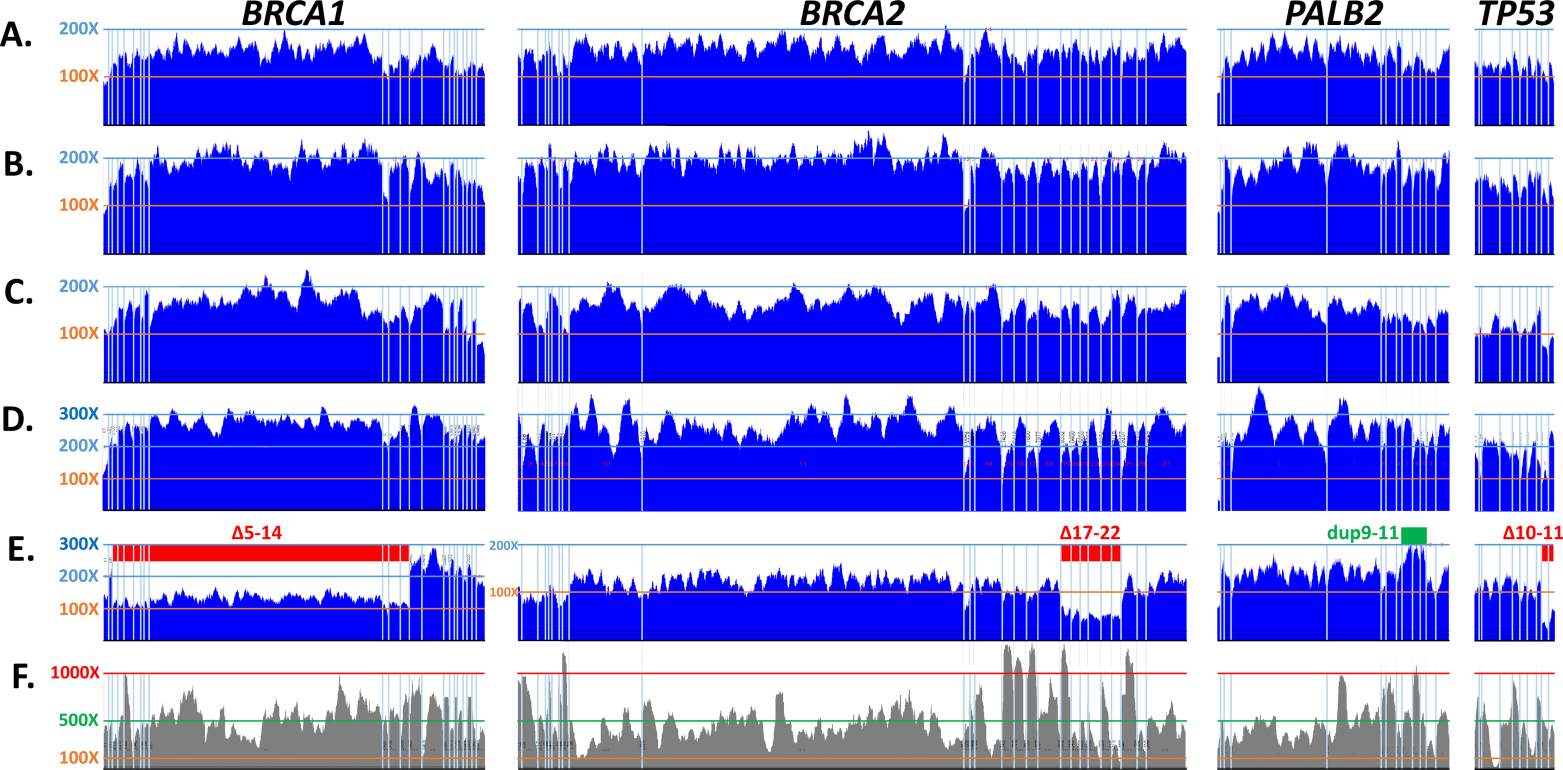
Coverage of selected genes from the CZECANCA (A-E) and TruSight Cancer sequencing (F) panels. The pictures show coverage (at y-axis) alongside the coding sequences of *BRCA1* (NM_007294), *BRCA2* (NM_000059), *PALB2* (NM_024675), and *TP53* (NM_000546), the vertical lines represent exon boundaries. Panels A–D show results obtained from a CZECANCA NGS analysis of various samples performed in four participating laboratories using the ultrasound (A, B) or enzymatic (C, D) DNA fragmentation protocol. Examples of the identified CNV aberrations in the depicted genes (deletions in *BRCA1*, *BRCA2* and *TP53* and duplication in *PALB2*) are shown in panel E. For comparison, panel F demonstrates the uneven coverage of the depicted genes by sequencing using the TruSight Cancer panel (Illumina).
